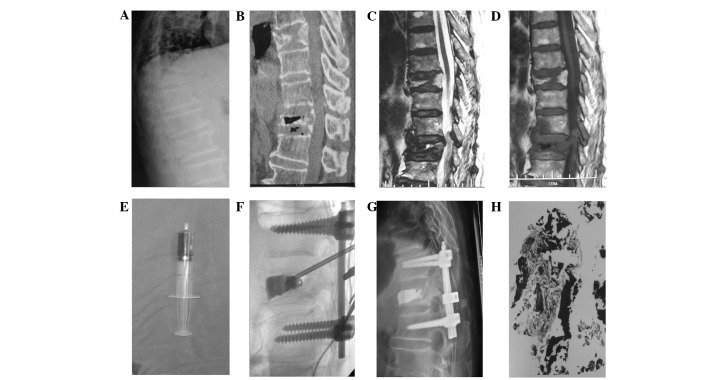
A 76-year-old male patient with bilateral lower limb numbness and weakness for half a year prior to admission and a preoperative BMD of −3.8 standard deviation (SD). (A) Preoperative standing lateral radiograph revealed T12 compression fracture and kyphosis. (B) Preoperative CT showed the intravertebral vacuum sign and gas in the adjacent disc. (C and D) Preoperative MRI displayed a mixed signal of liquid and gas within the vertebral body. (E) Liquid collected intraoperatively from the vertebral body. (F) Intraoperatively, the vertebral height was restored and the bone cement filled the cleft well with no leakage. (G) Standing lateral radiographs showed no decrease in vertebral height and no kyphosis recurrence after a follow-up of 18 months. (H) Postoperative pathology revealed necrosis of the bone. BMD, bone mineral density.
